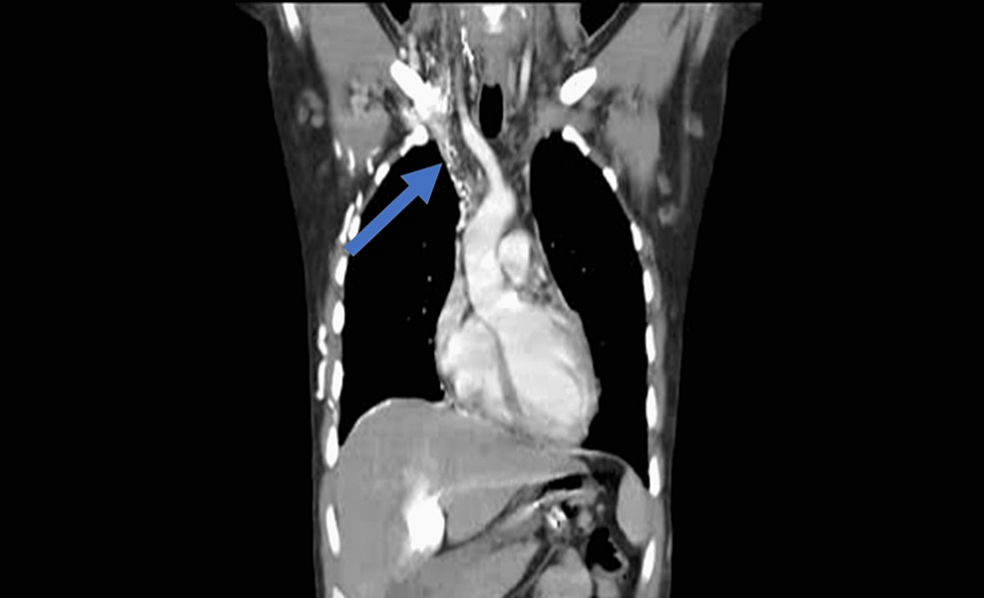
CT chest showing SVC thrombosis SVC: superior vena cava
